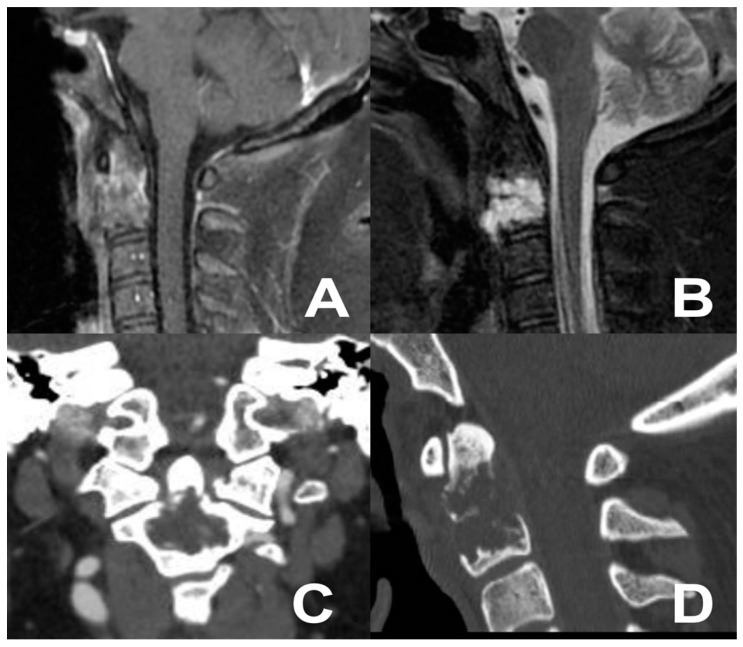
Cervical MRI (A,B) and CT scan (C,D) showing the osteolytic lesion of the C2 vertebral body.
